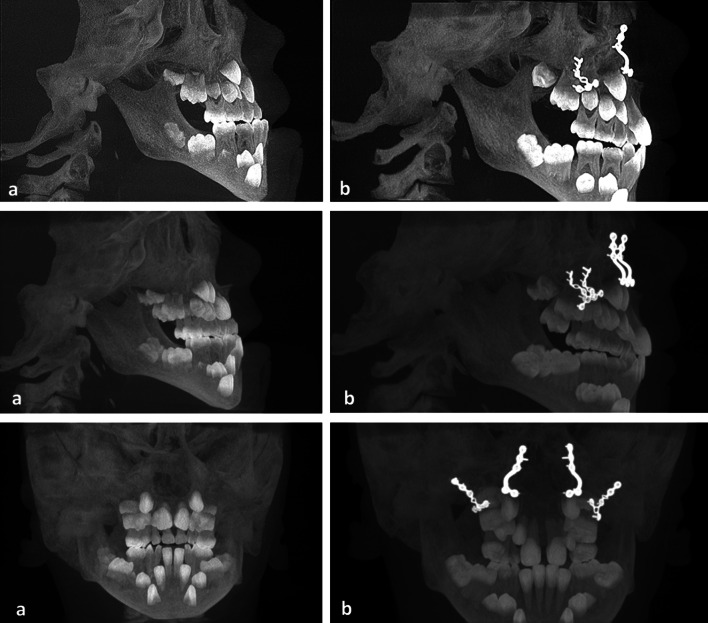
a X-ray images generated from a cbct scan 1 month and 7 days preoperatively. b X-ray images generated from a cbct scan 1 year and 5 months postoperatively
